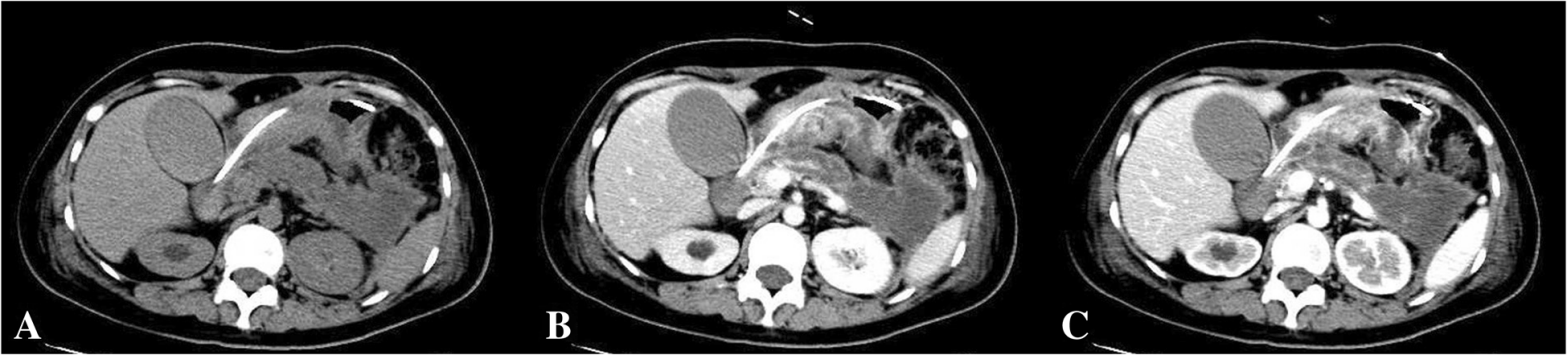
Abdominal CT scan. It showed diffuse enlarged pancreas with indistinct boundaries and surrounding exudates (a: non-contrast-enhanced image; b: arterial phase image; c: delayed phase image). CT = computed tomography
